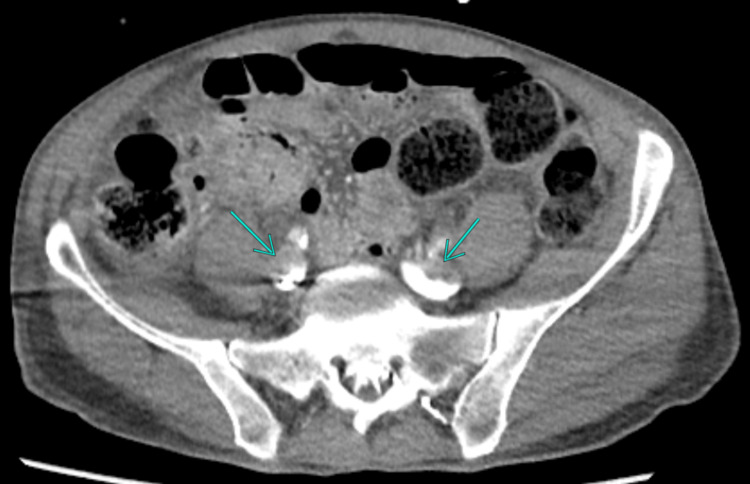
Portal-phase CT angiography of the abdomen demonstrating venous thrombi in the common iliac veins
